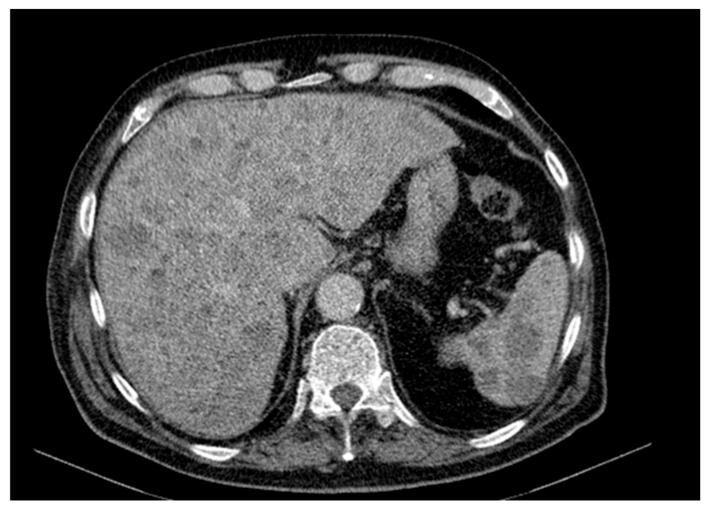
Contrast-enhanced abdominal and pelvic CT scan revealing hepatosplenomegaly with multiple hypodense, non-enhancing nodular lesions suggestive of secondary involvement. Bilateral renal cysts, more numerous and larger on the right (up to 50 mm), a 9 mm right renal pelvis stone, and the presence of a right ureteral stent are noted. Diffuse bone structure changes with osteoporotic background and poorly defined hyperdensities are seen, along with L1 vertebral body collapse.
